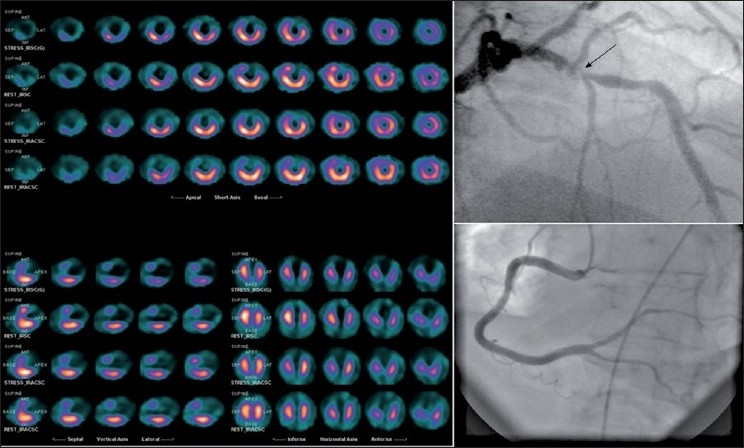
Myocardial perfusion scintigraphy showing an extensive fixed perfusion defect in the LAD territory, corresponding with the significant stenosis of the LAD on invasive angiography, (arrow). Revascularization was deferred in this patient because of the absence of a significant viable myocardium in the involved territory
